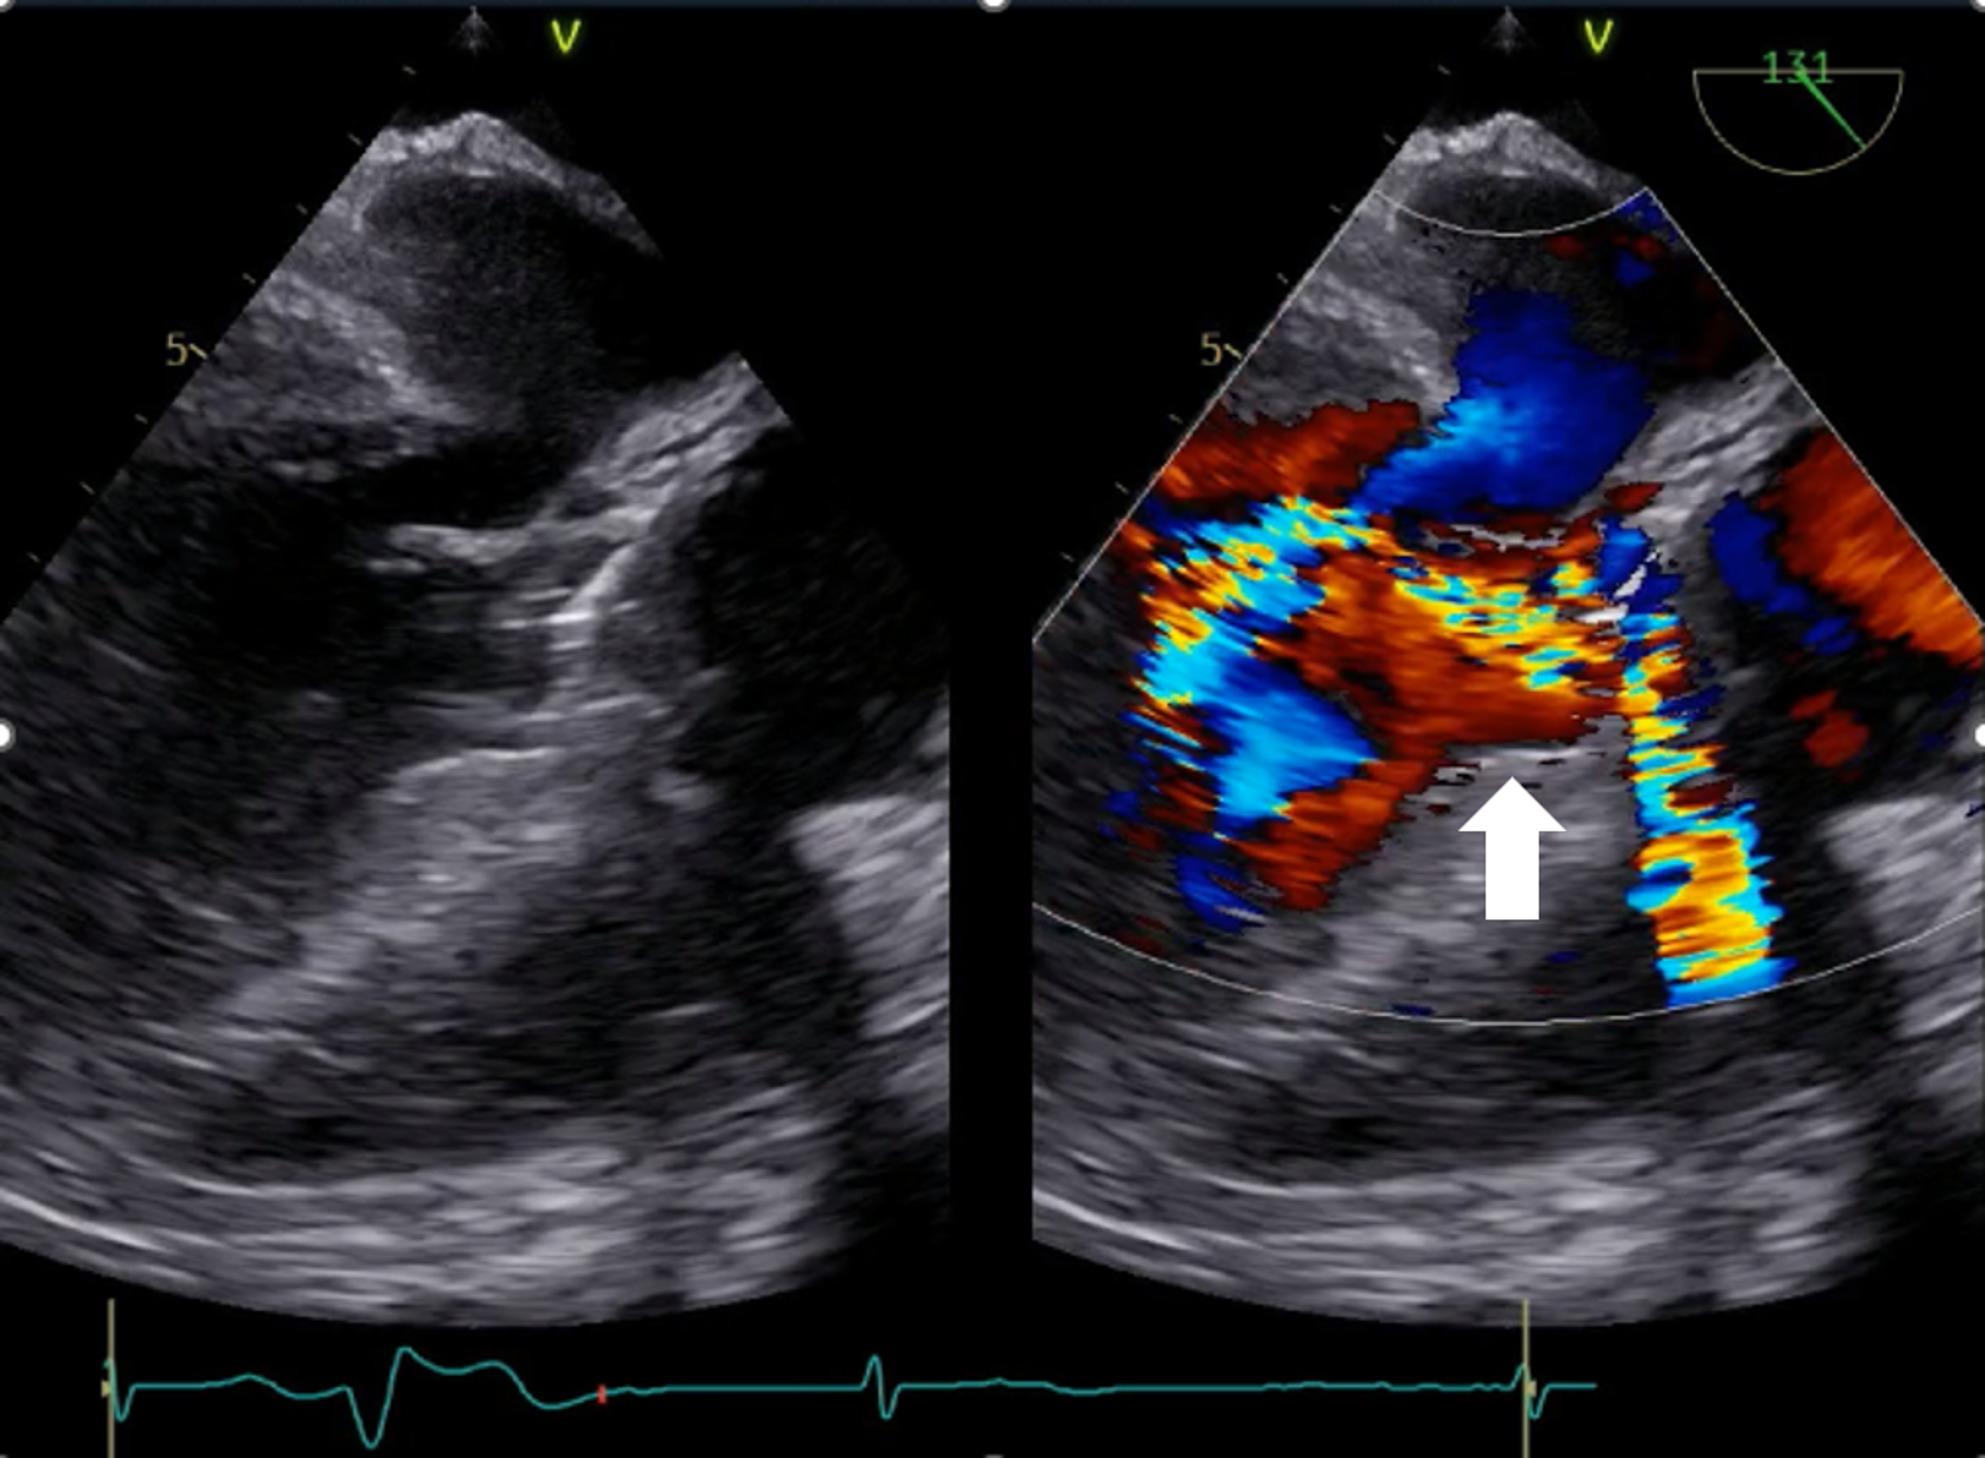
Transesophageal echocardiography showing paravalvular regurgitation surrounding the aortic valve prosthesis Preoperative transesophageal echocardiography revealing severe regurgitation around the prosthetic valve in the position of the aortic valve (white arrow)
